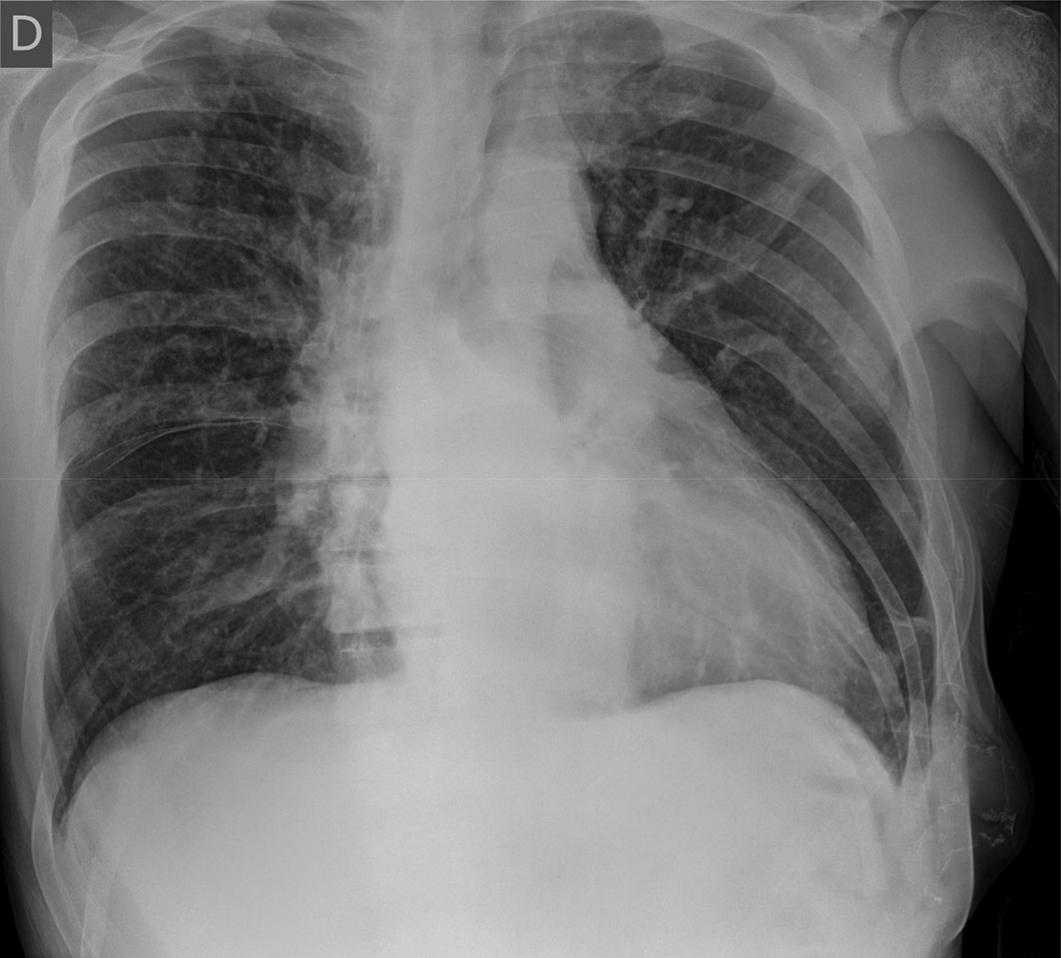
Chest radiograph at hospital admission.
